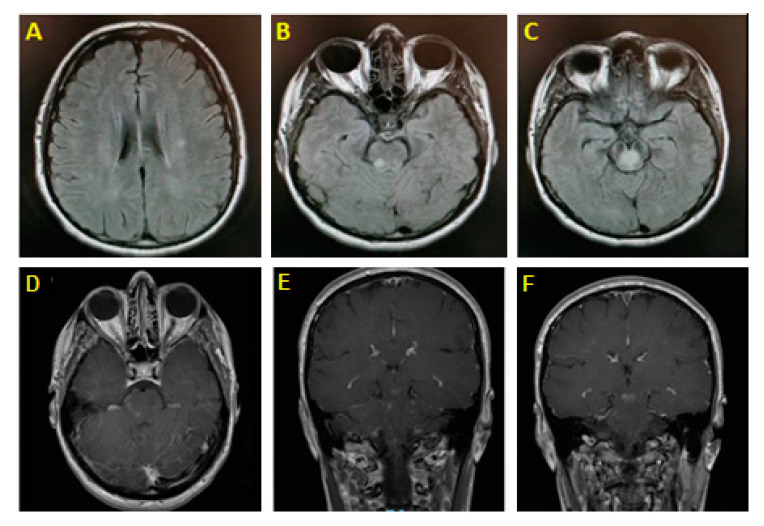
(A): Axial T2/FLAIR shows bilateral foci signal hyperintensities in the subcortical white matter. (B): Right pontine T2/FLAIR hyperintensity. (C): Midbrain hyperintense signal. (D–F): Subcortical lesions showing postcontrast enhancement.
